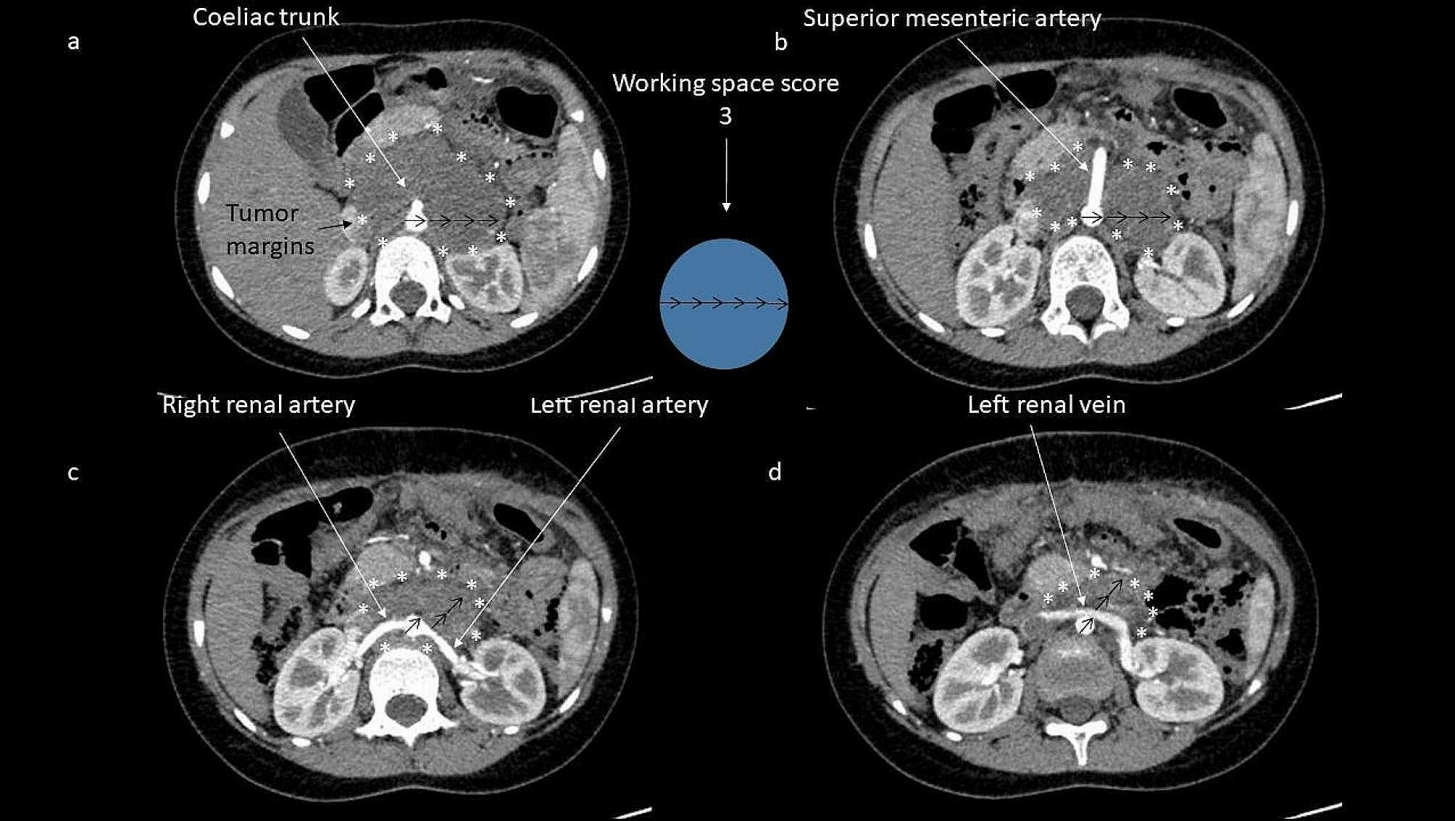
Preoperative computed tomography after application of intravenous contrast substance of a child following induction chemotherapy with persistent vascular encasement of major abdominal arteries by neuroblastoma Borders of the tumor (starlets) and expected working space as a multiple of the aortal diameter (arrows) required to see the tumor margin at the level of the (a) coeliac trunk, (b) superior mesenteric artery, (c) right renal artery, (d) left renal vein. The circle depicts the desired circular maximum working space from the center of the landmark vessel equaling a radius of 3x the diameter of the aorta (working space score 3)
